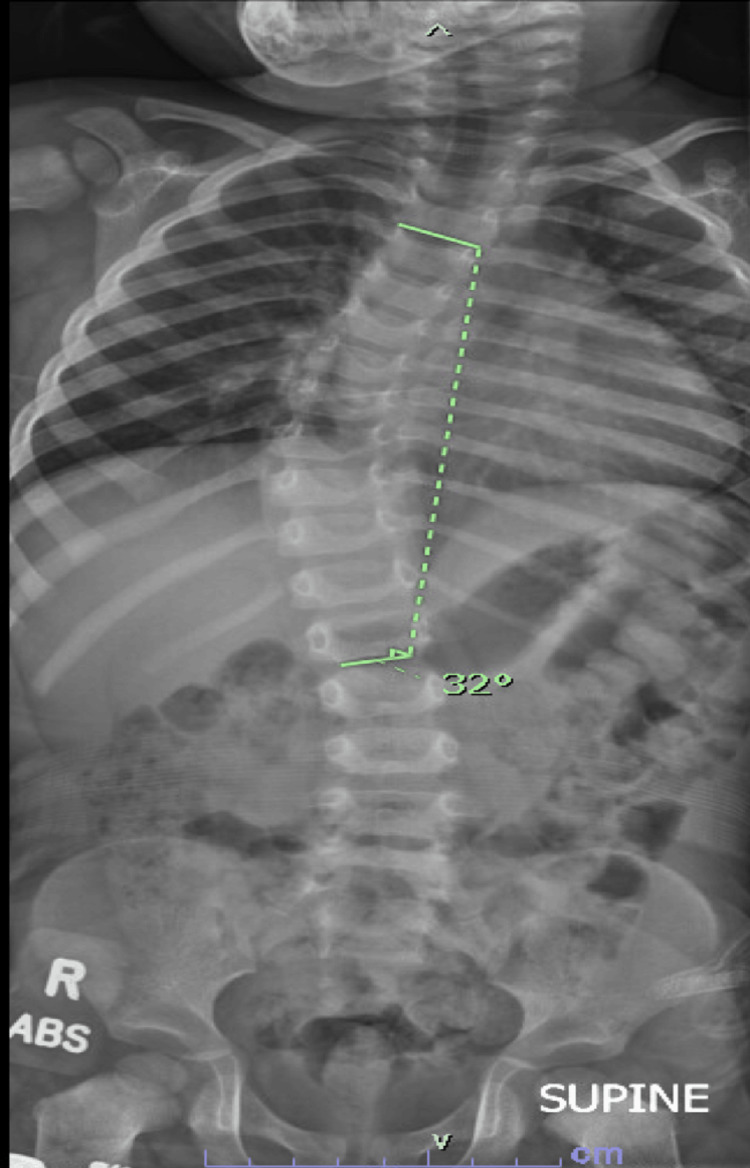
Spine anterior-posterior X-ray for scoliosis There is moderate dextroscoliosis of the thoracolumbar spine. This measures about 32 degrees and features moderate rotatory scoliosis tearing.
